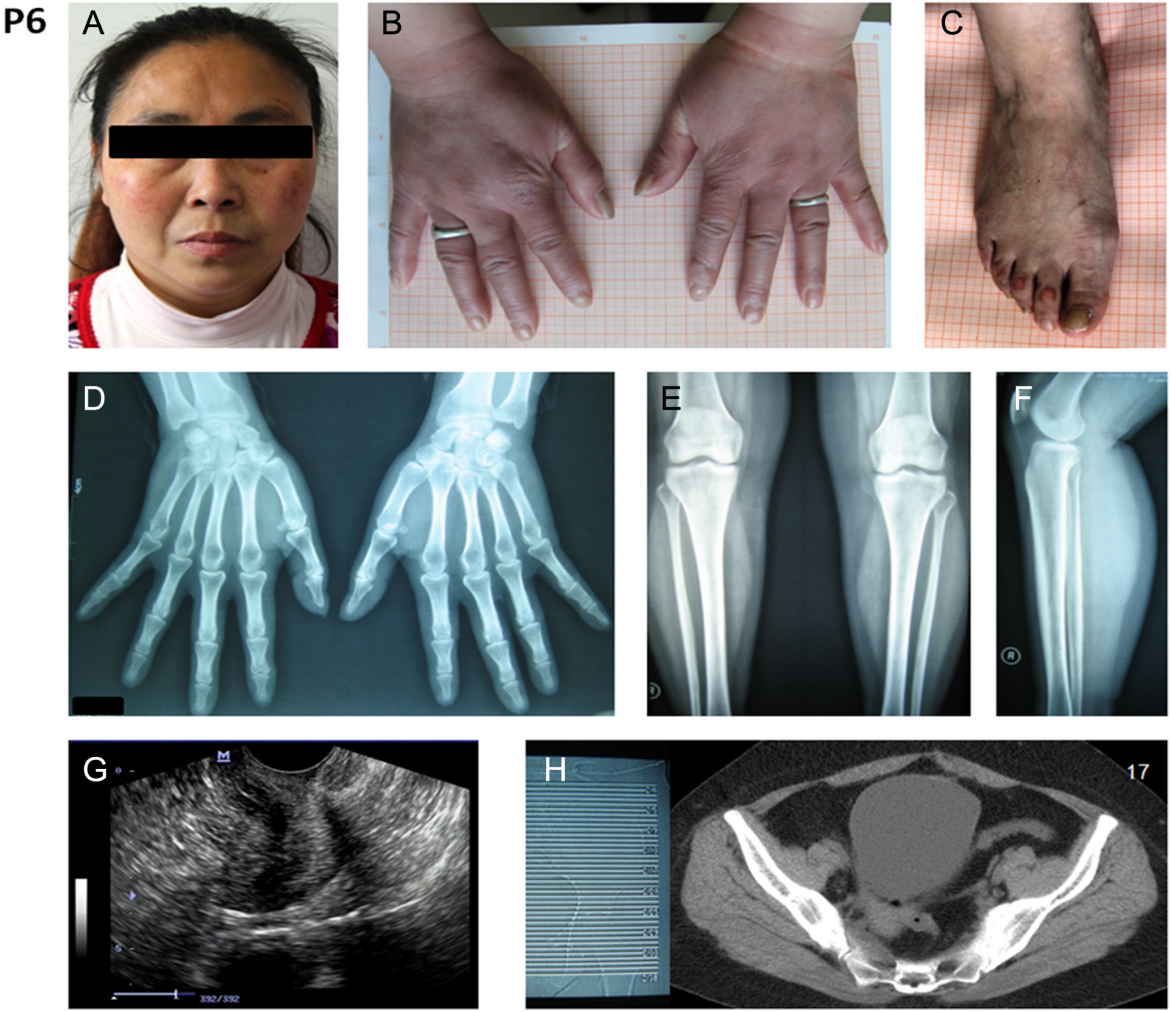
Clinical images of the unaffected individual: Family4, II.3-P6. The images showed no thickening and furrowing of facial skin; and the clubbing of fingernails or toenails (A, B and C). A radiograph of tibiofibula, hands and feet show no sign of periosteal hyperostosis (D, E and F). Gynecological B-ultrasound examination showed invisibility of both ovaries (G). CT screen is also hard to detect both ovaries (H). All images are published with permission from the individual.
